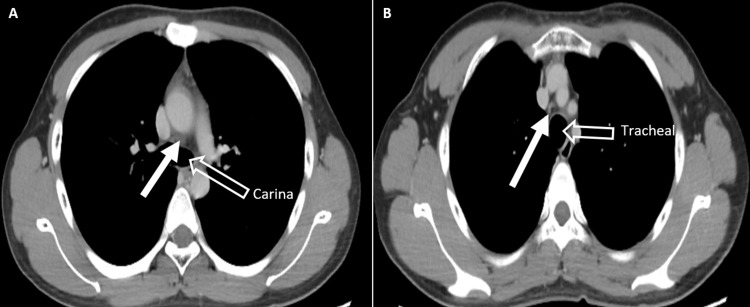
Contrast-enhanced axial CT of the thorax Contrast-enhanced images of the thorax demonstrate non-necrotizing lymph nodes (white arrow) seen in the (A) subcarinal and (B) paratracheal.
